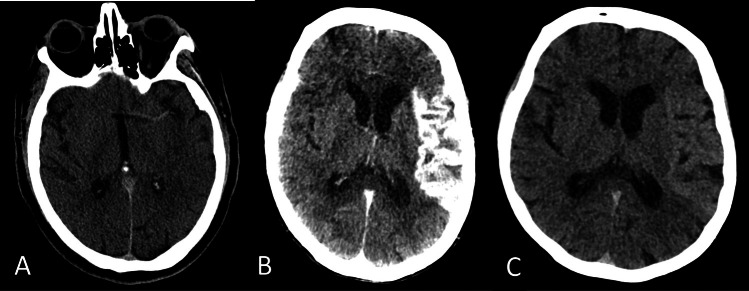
A. Non-contrast head computed tomography (CT) demonstrates a linear hyperdensity at the expected location of the left middle cerebral artery (MCA) consistent with acute thrombus, "hyperdense MCA" sign. B. Dual Energy head CT in a patient with left MCA territory stroke, status post endovascular intervention. Mixed image (left) shows gyriform pattern of intense hyper-attenuation involving the left insular and left temporal-frontal-parietal cortices. Virtual unenhanced image (right) shows mild hyper-attenuation and gray-white matter differentiation loss of the left MCA territory which is suggestive of blood brain barrier breakdown, neovascularization and impaired autoregulation. There was no hemorrhagic transformation
